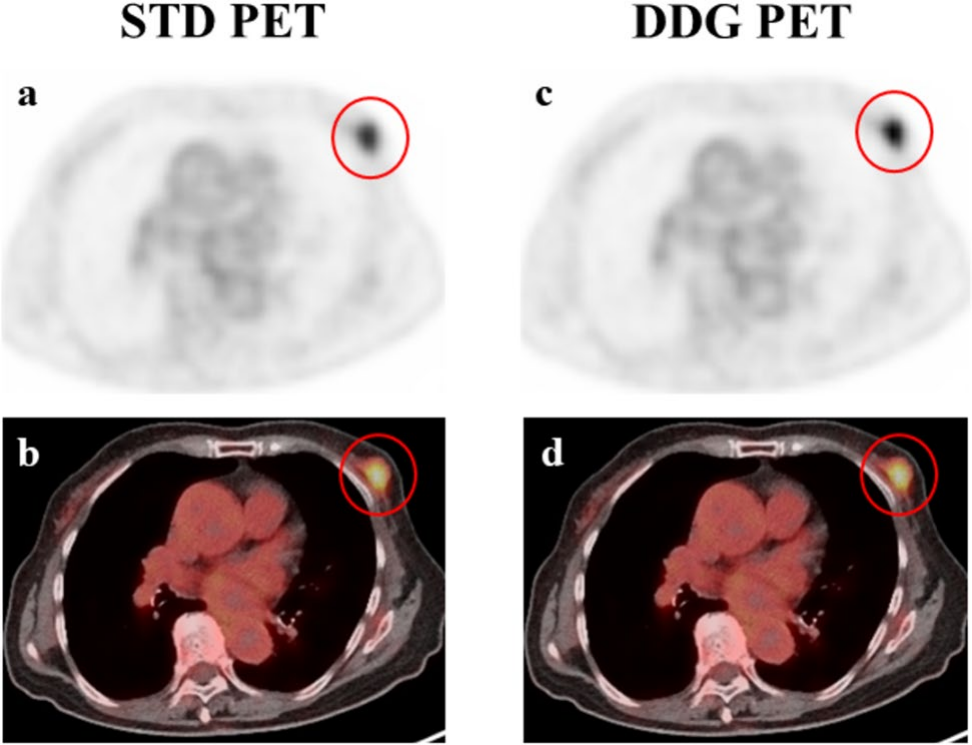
Left breast cancer. The left breast cancer lesion was visualized with less blurring in DDG than in STD FDG PET. SUVmax and MTV in DDG (5.9 and 2.9 ml) were higher and lower, respectively, than those (4.9 and 3.9 ml) in STD PET. *STD*: standard free-breathing, *DDG*: data-driven respiratory gating. a, c: Transaxial FDG PET image, b, d: fused FDG PET/CT image
